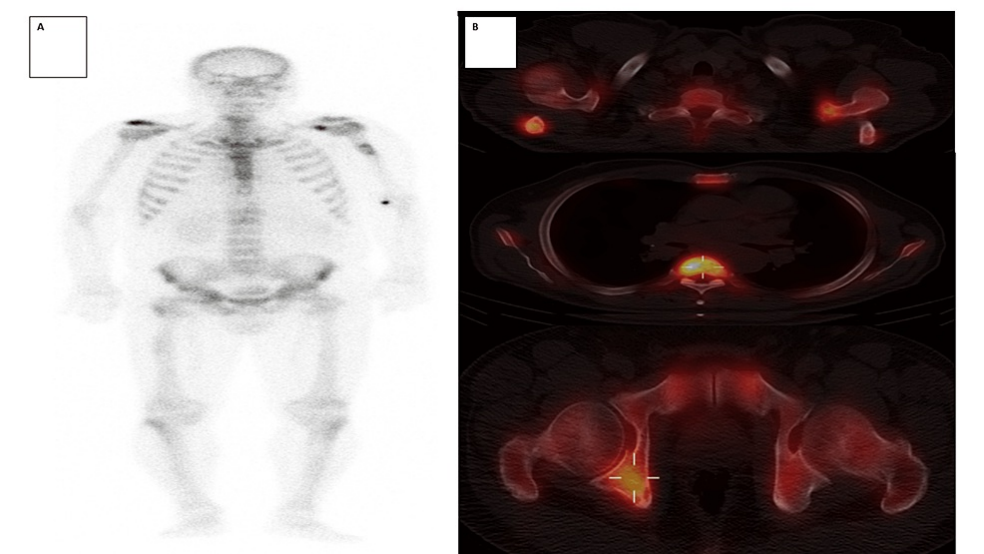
Bone scan with 99mTc-MDP. A) Whole body scan in anterior and posterior projection and SPECT/CT. High-uptake bone lesions are observed in the scapulae, proximal third of the left humerus, B) vertebral body of T5, eleventh posterior left costal arch, right ischium, and greater trochanter of the right femur secondary to metastatic involvement. MDP: methylene diphosphonate
